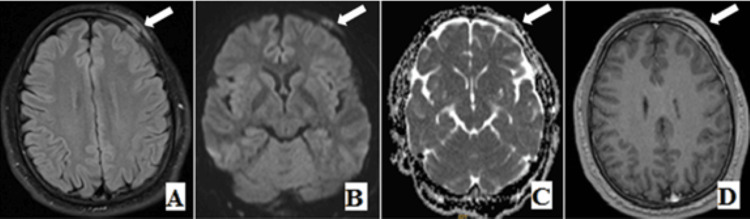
Axial T2/FLAIR, axial DWI/ADC Map (B,C), and axial gadolinium enhanced T1(D) showing T2/FLAIR hyperintense signal of the skull lesion in the left frontal bone and T2/FLAIR hyperintense soft tissue correlated with restriction on diffusion and enhancing bone, underlying meninges and overlying soft tissue (white arrow). DWI: Diffusion-weighted imaging, ADC: Apparent diffusion coefficient
